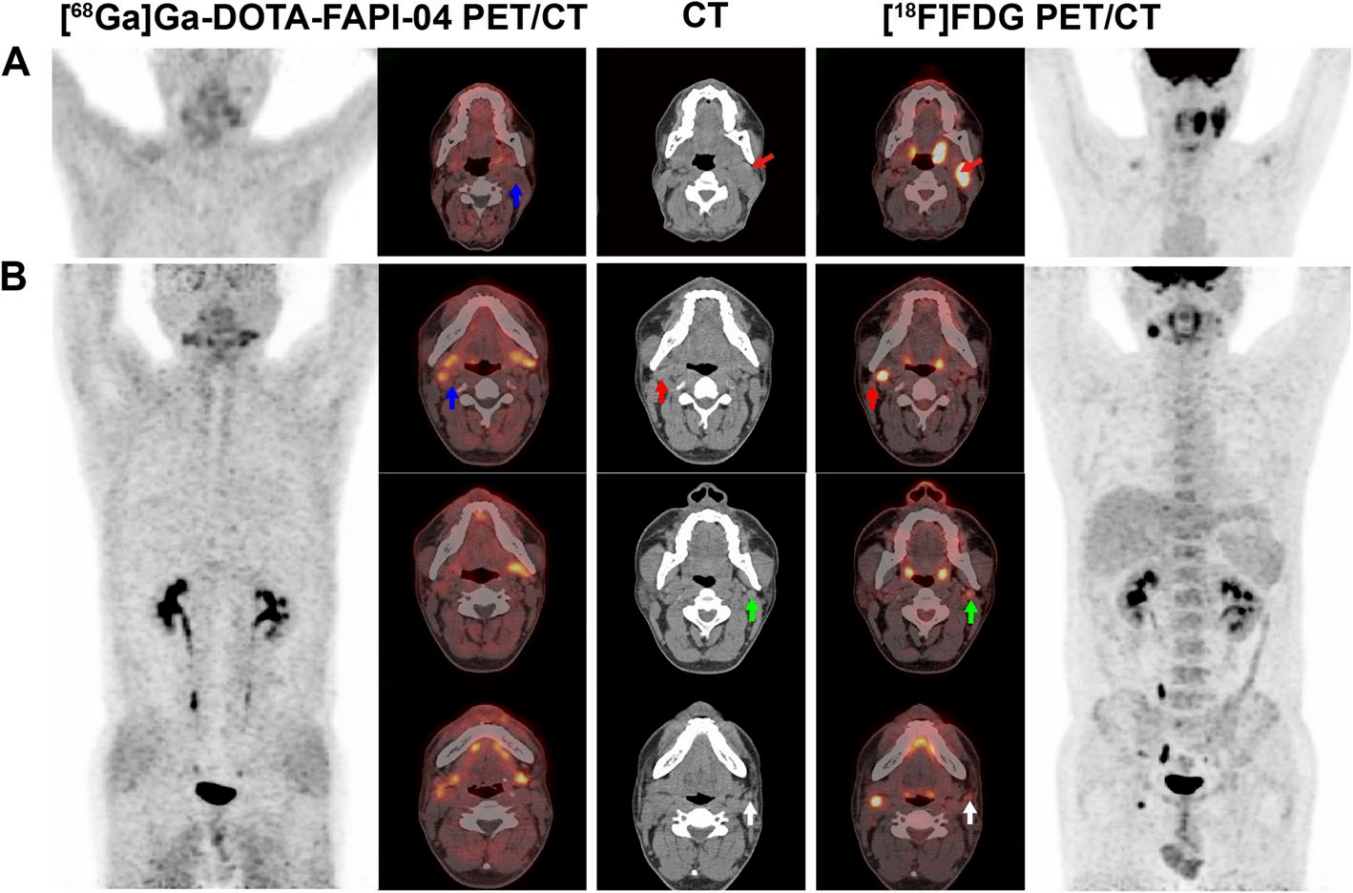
A A 60-year-old man with tonsil cancer accompanying metastatic squamous carcinoma of the left neck. CT and [^18^F]FDG PET/CT showed positive lymph nodes on the left side level IIa (red arrow; SUVmax 7.8). [^68^ Ga]Ga-DOTA-FAPI-04 PET/CT showed low uptake of lymph nodes on the left side level IIa (blue arrow; SUVmax 2.5). Metastasis was pathologically confirmed metastasis. B A 39-year-old man with tonsil cancer associated with metastatic squamous carcinoma of the right neck. [^18^F]FDG PET/CT detected one lymph node on the right side at level IIa (red arrows; SUVmax 4.8) and two lymph nodes on the left side at level IIa (green arrows; SUVmax 4.2; white arrows; SUVmax 2.9), while [^68^ Ga]Ga-DOTA-FAPI-04 PET/CT detected only one lymph node on the right side at level IIa (blue arrow; SUVmax 12.6). Based on the pathological diagnosis and clinical follow-up, only the right level IIa node was identified as a lymph node metastasis
